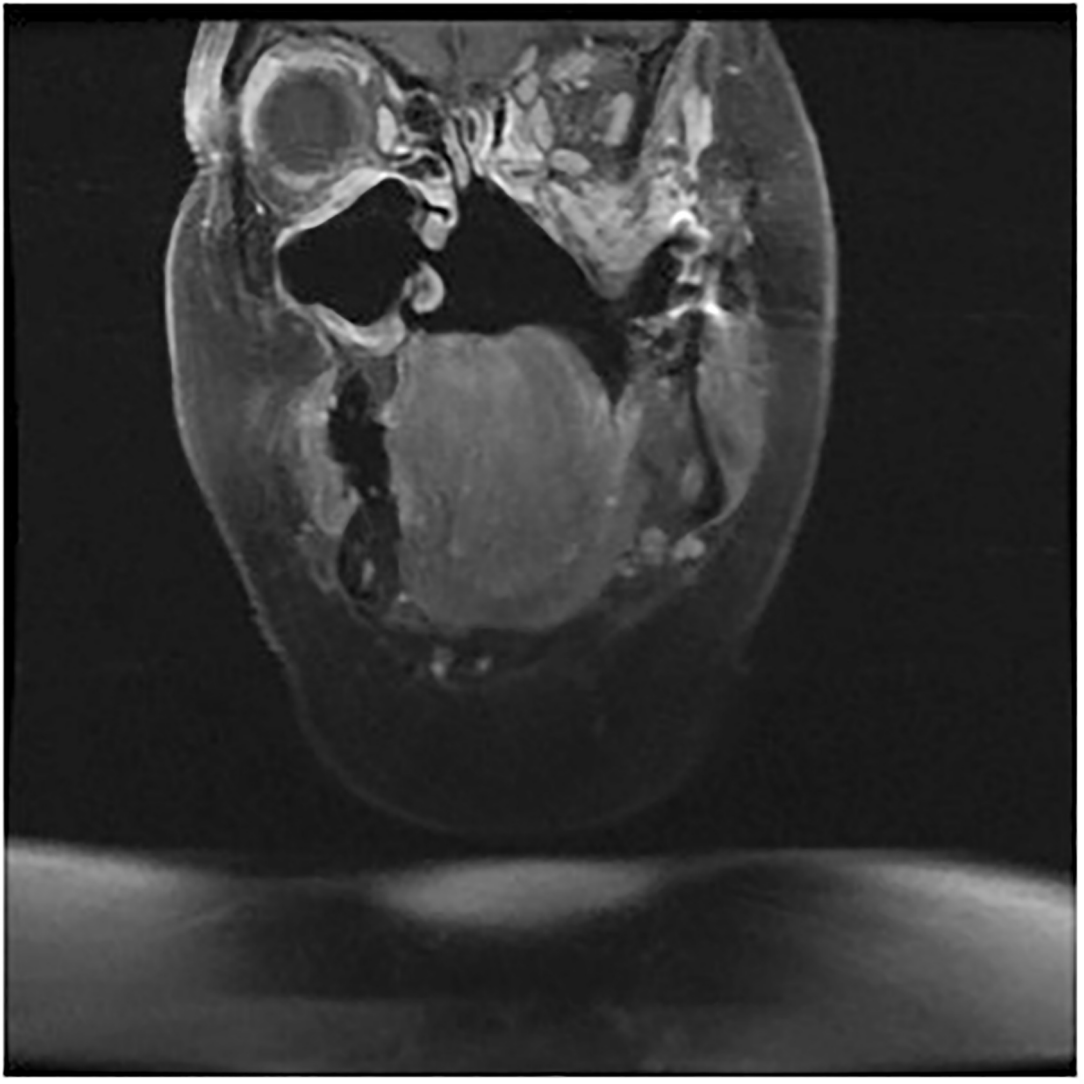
Coronal CT scan showing recurrence of tumour involving left masticator space.
